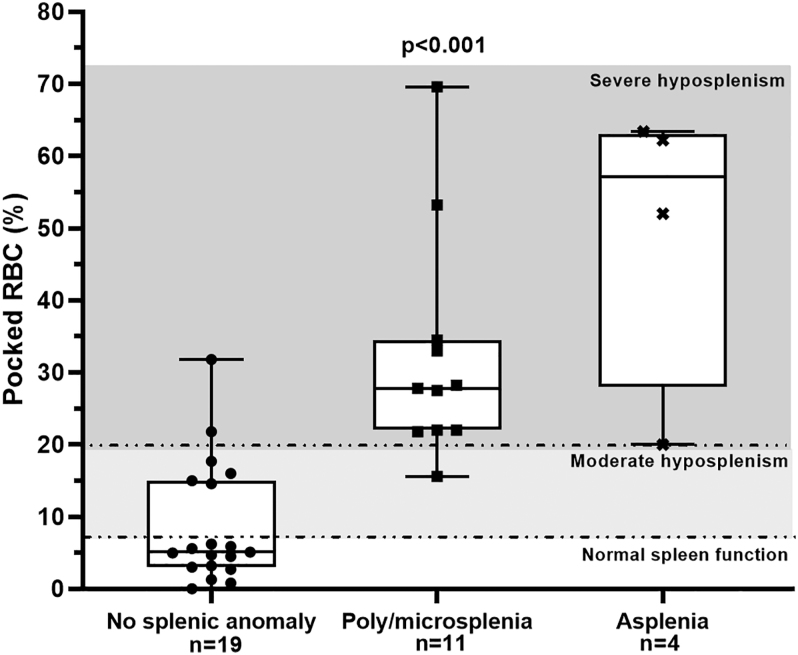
Pocked RBCs according to spleen morphology in *NR5A1* patients. Asplenia was defined by an absent or atrophic spleen – normal spleen function (white zone) was defined by a pRBC <7%, moderate hyposplenism (light grey zone) by a pRBC between 7 and 20%, and severe hyposplenism (dark grey zone) by a pRBC >20%. Spleen morphology was determined by ultrasound, CT scan, or MRI. *n*: number of patients; RBC: red blood cell. A Jonckheere-Terpstra test was performed (the Jonckheere–Terpstra test assessed an ordered trend across spleen-morphology categories with the *a priori* order ‘normal < micro/polysplenia < asplenia’).
